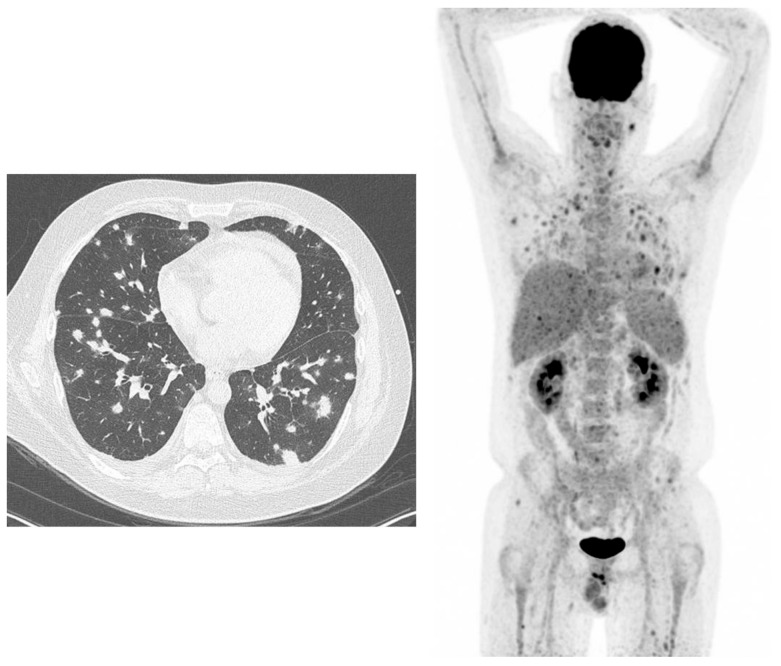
38-year-old male, known with acute lymphatic leukaemia. Because of fever and bacteraemia he was treated with meropenem (antibiotics). High-resolution computed tomography (HR-CT) (left figure): several lung densities with irregular borders, not typical for bacterial infections, but typical for angio-invasive aspergillosis, because of the halo sign. ^18^F-fluorodeoxyglucose positron emission tomography/computed tomography (^18^F-FDG-PET) (right figure): not only lung involvement but also infectious lesions in the liver, spleen and in muscles throughout the body.
